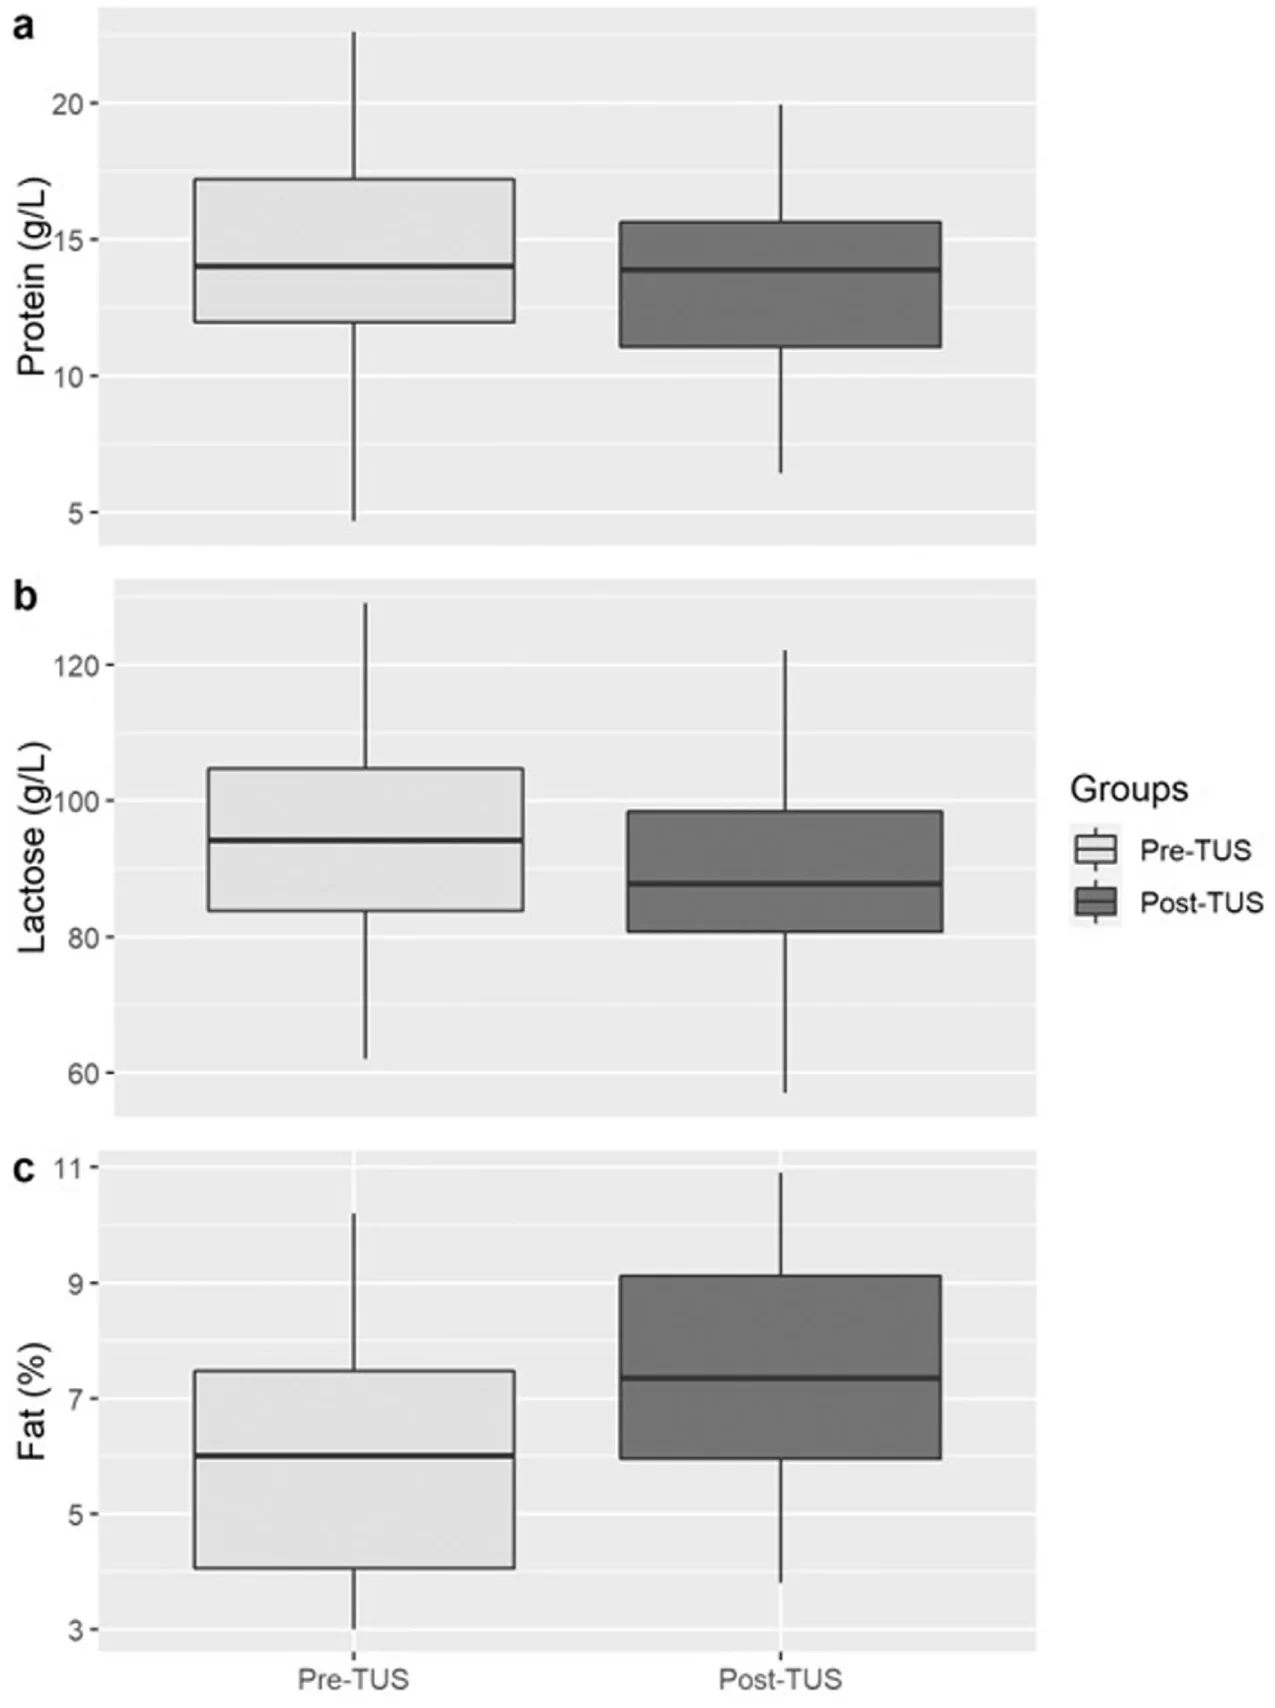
Box Plot Graphs Showing Concentrations of (a) Protein, (b) Lactose and (c) Fat Pre and Post Therapeutic Ultrasound. *Note.* Pre-TUS = pre application of therapeutic ultrasound; Post-TUS = post application of therapeutic ultrasound; g/L= grams per litre.
